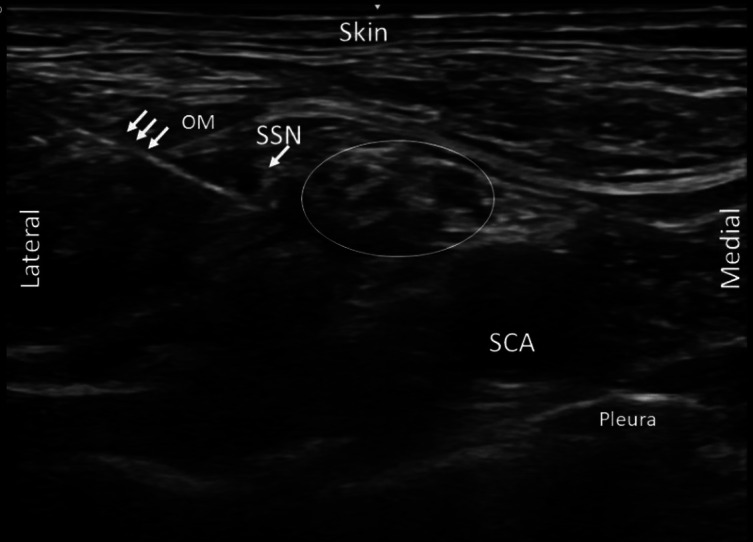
Ultrasonographic image of the supraclavicular fossa with the transducer positioned in an oblique sagittal plane. OM, omohyoid muscle (triple arrow); SCA, subclavian artery; SSN, suprascapular nerve (single arrow); needle and encircled is the brachial plexus.
